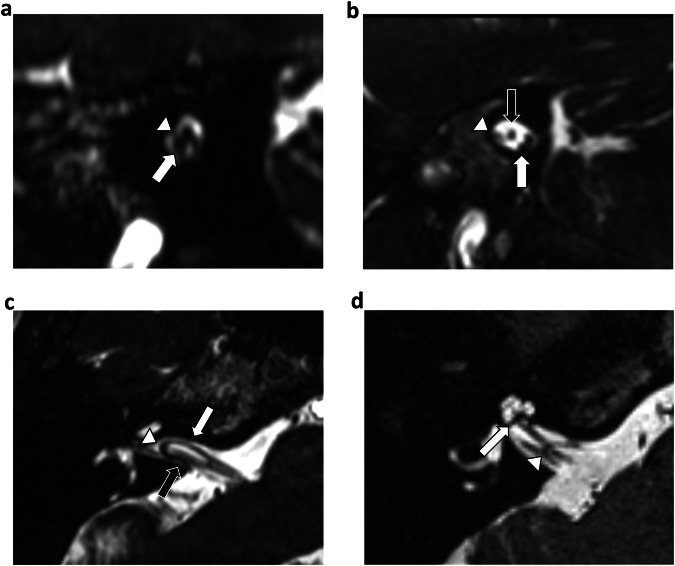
Image analysis for NVC and continuous variables. a NVC (sagittal-oblique reformatted unenhanced T2w CISS image). NVC was identified as contact between the VL and the vestibulocochlear or cochlear nerve within the CPA or IAM without a visible intervening normal cerebrospinal fluid signal. The image shows NVC of an IVL (arrowhead) with the cochlear nerve inferiorly (white arrow). b An example of the absence of vestibulocochlear NVC within the IAM (sagittal-oblique reformatted unenhanced T2w CISS image). The IAM VL (arrowhead) is located anterior to the facial nerve (black arrow), with normal intervening cerebrospinal fluid signal separating the VL from the cochlear nerve (white arrow) located postero-inferiorly. c Loop interlimb distance (axial unenhanced T2w CISS image). Distance (mm) was measured between the proximal (black arrow) and distal (white arrow) limbs of the VL at 2 mm from the VL apex (arrowhead). d Loop-fundus distance (axial unenhanced T2w CISS image). Distance (mm) was measured from the VL apex (arrowhead) to the base of the modiolus (arrow). CISS, constructive interference in steady state; CPA, cerebellopontine angle; IAM, internal auditory meatus; NVC, neurovascular contact; T2w, T2-weighted; VL, vascular loop
